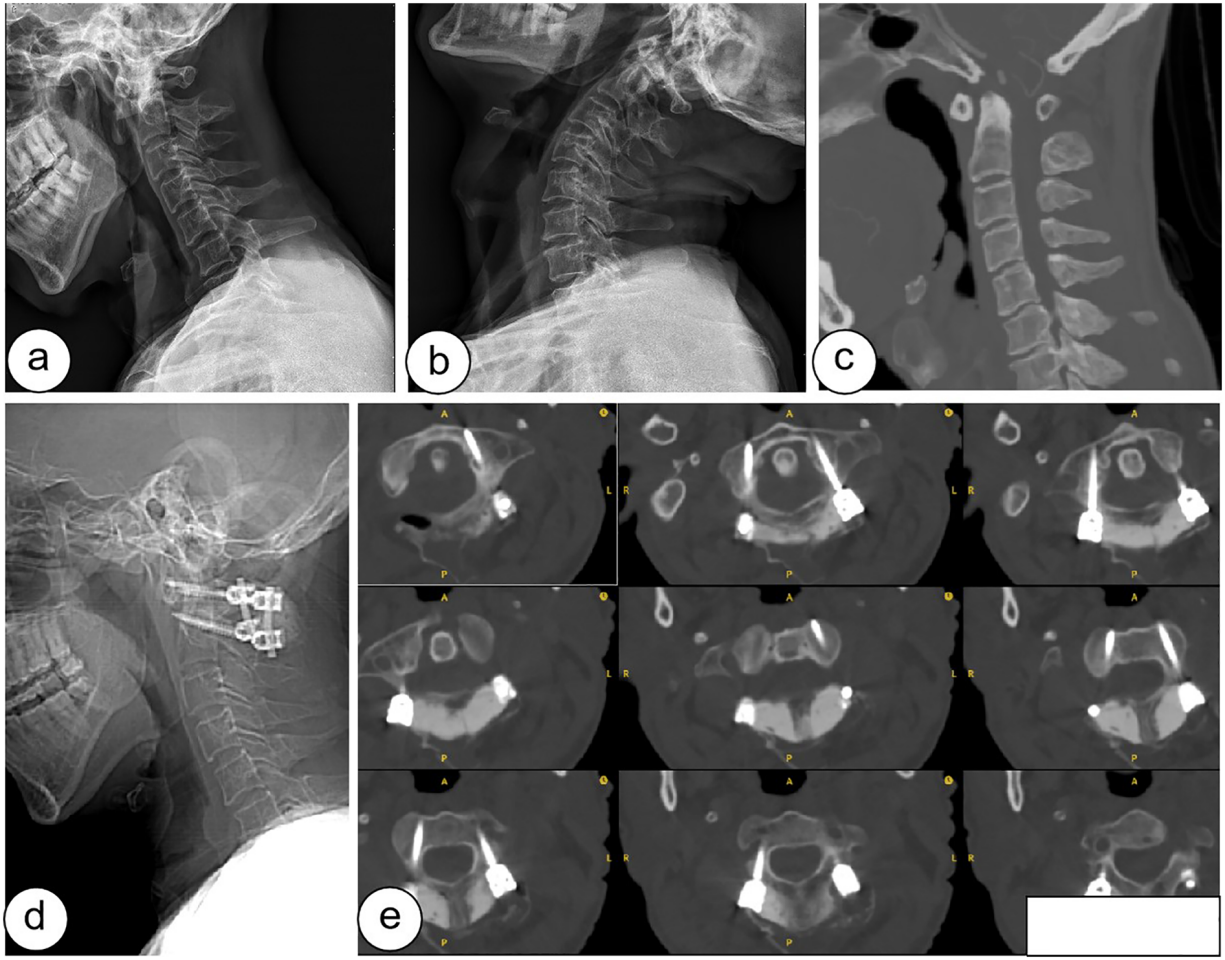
Preoperative and postoperative images of the patient: (a-c) are preoperative hyperextension, hyperflexion and CT of the cervical spine, (d-e) are lateral and CT of the cervical spine after surgery.
